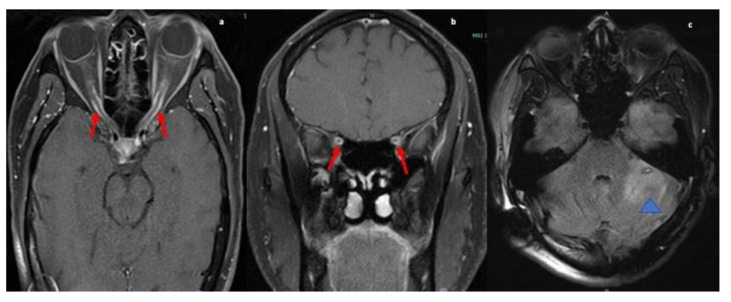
MRI orbit axial T1-weighted fat-suppressed post contrast image (a) and coronal (b) images reveal enhancement of bilateral optic nerve sheaths (red arrow). MRI Brain axial fluid-attenuated inversion recovery (FLAIR) (c) image reveals ill-defined patchy parenchymal hyperintensity in the left cerebellar hemisphere extending to the cerebellar peduncle (blue arrowhead).
